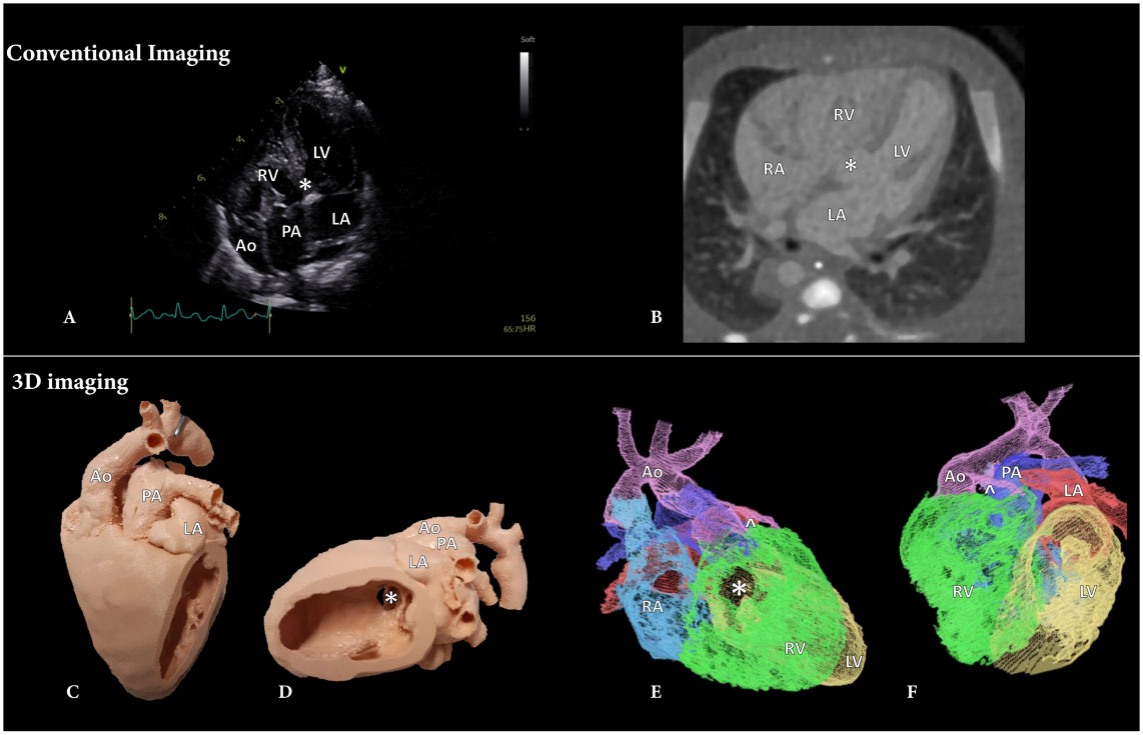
Imaging modalities used in double outlet right ventricle patients. (A) Ultrasound 4-chamber overview, (B) axial CT image, (C) anterior view of the 3D printed model, (D) lateral view of the 3D printed model, (E) right lateral view of the 3D-VR reconstruction, (F) anterior view of the 3D-VR reconstruction. Ao: aorta; LA: left atrium; LV: left ventricle; PA: pulmonary artery; RA: right atrium; RV: right ventricle; ^: coronary artery; *: ventricular septum defect.
